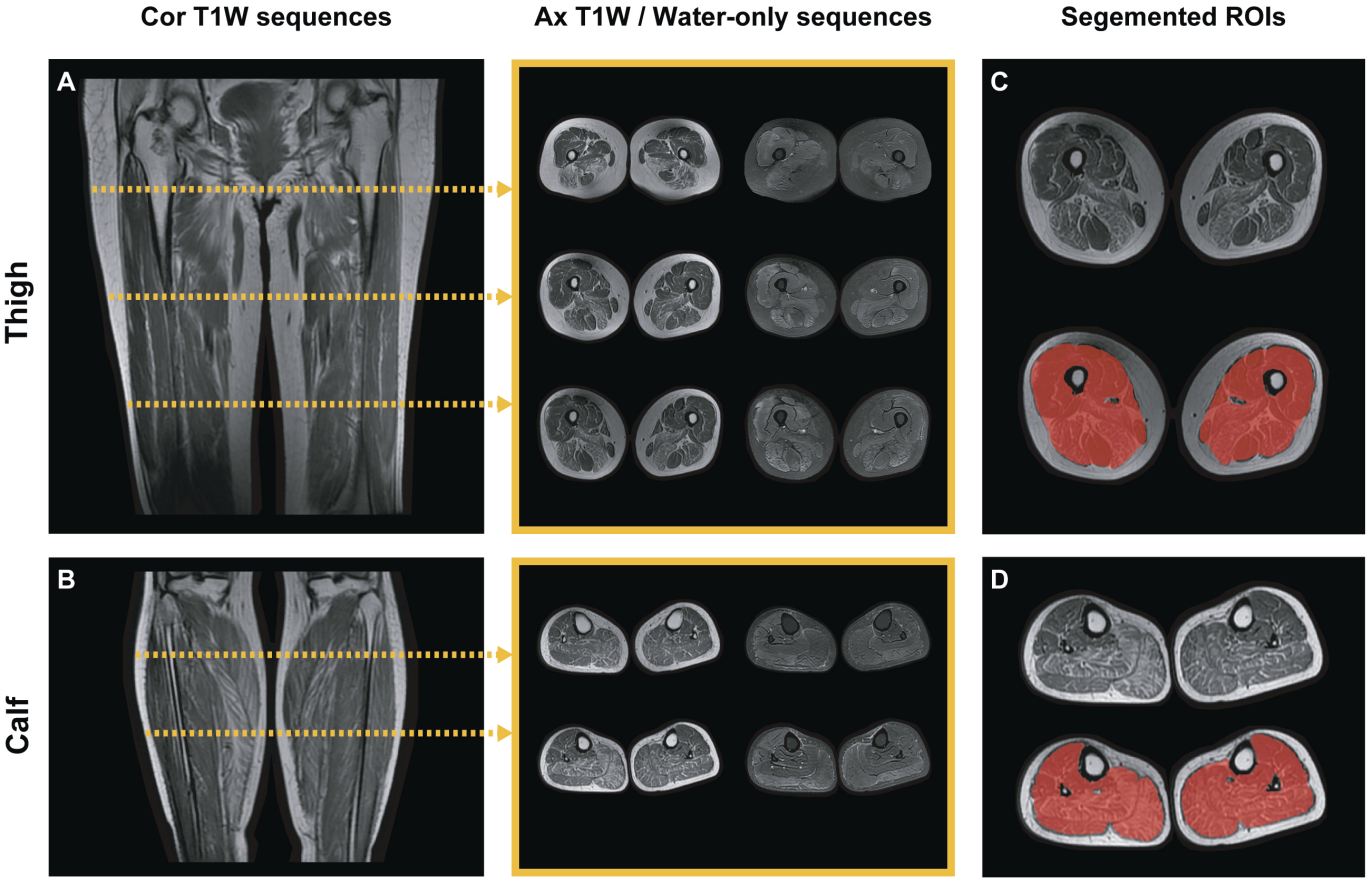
Lower-limb muscle MR image selection and segmentation. (A,B) Two images showed the coronal T1-weighted calf and thigh images. For each patient, five slices in different areas were selected for radiomics analysis: three slices of thighs and two slices of calves for each sequence (T1-weighted and water-only). The middle showed the selected slices of axial T1-weighted and water-only images, respectively. (C,D) The global area of the muscles in each selected slice was segmented as calf or thigh ROI (red-shaded area), excluding the epimysium.
